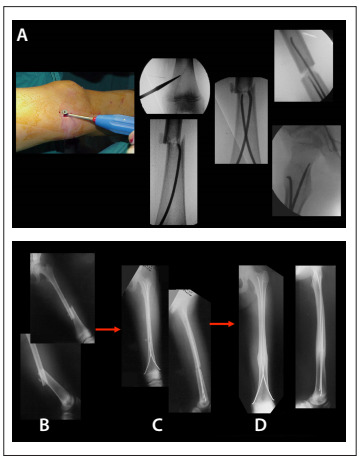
Fixation of flexible intramedullary titanium elastic nails (TEN) in a child with femoral shaft fracture: procedure on a radiotransparent table, with the patient in supine position (A) and radiographs taken initially (B), in the immediate postoperative period (C) and finally (D).
